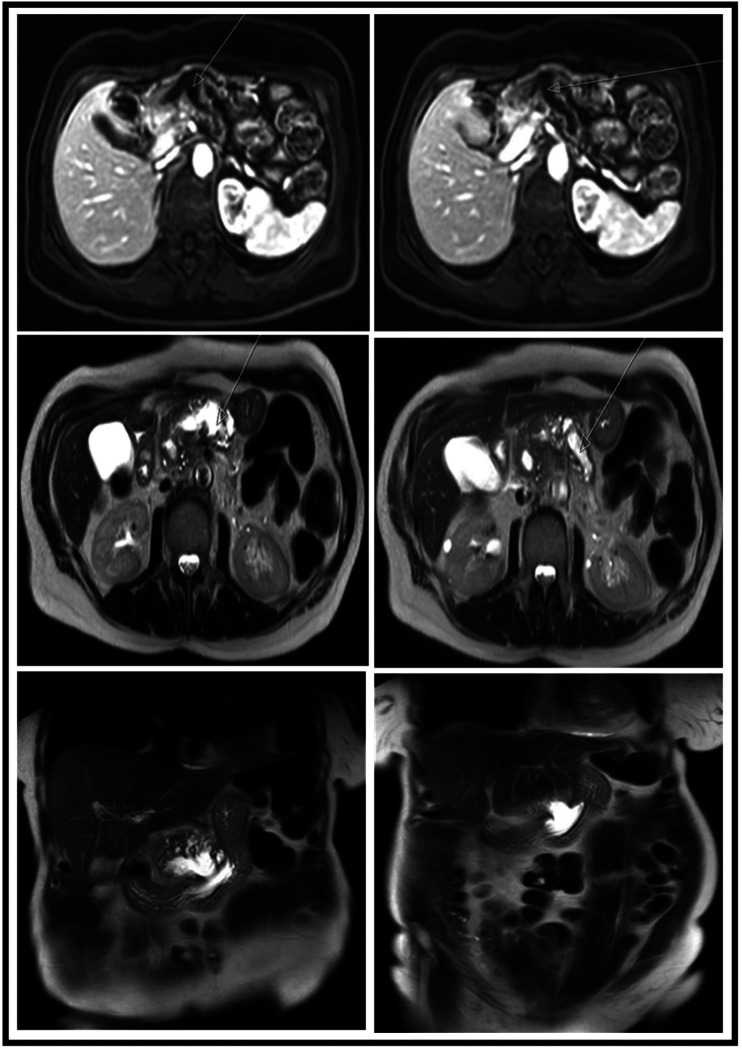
MRI images showing different views and sequences of the fistulation between the MPD and the stomach. Upper row, axial T1 + gadolinium; middle row, axial T2-weighted images; lower row, coronal T2-weighted images. MPD, main pancreatic duct.
